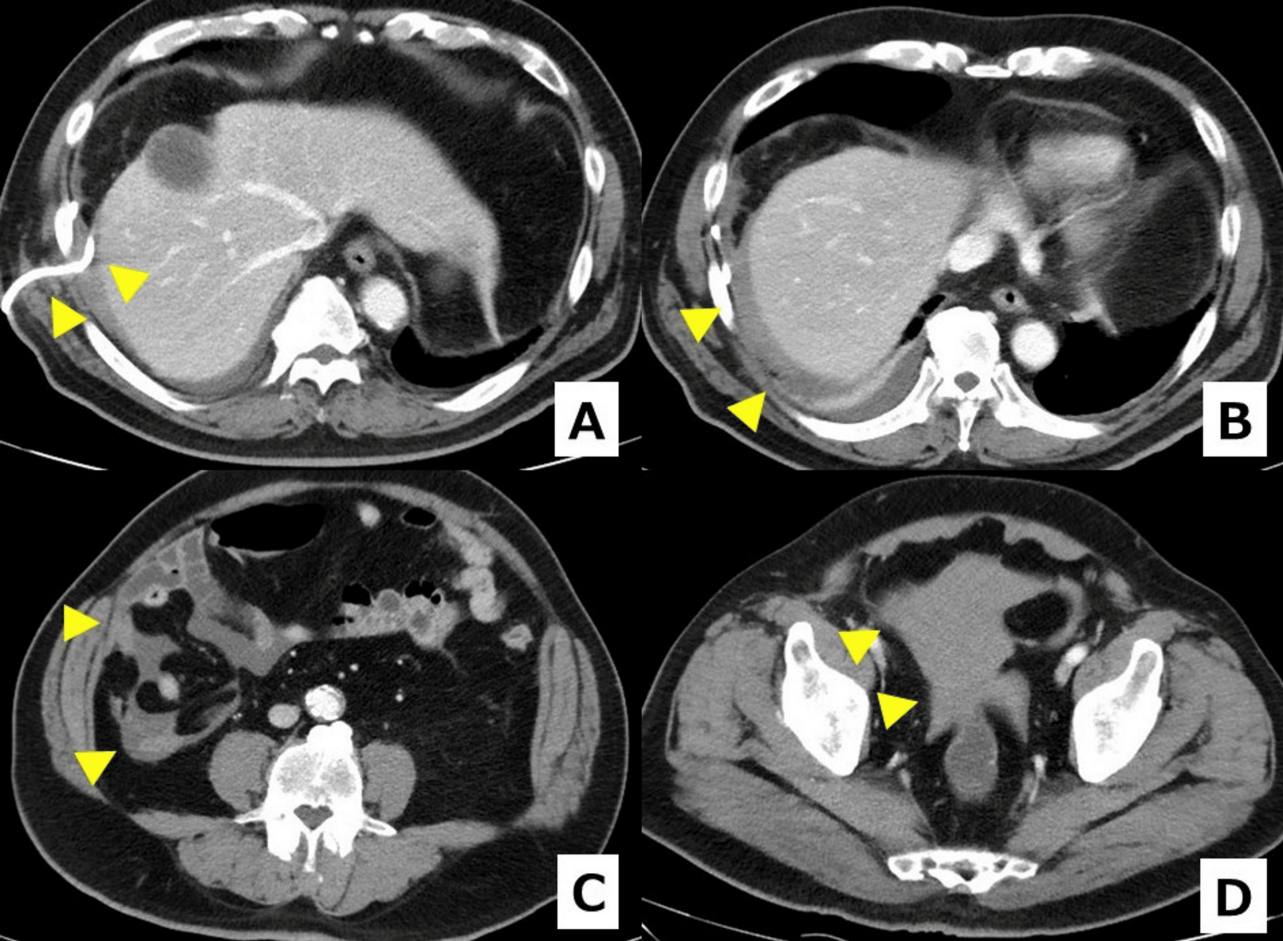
Contrast-enhanced CT after spontaneous PTGBD tube dislodgement (A) Arrowhead: PTGBD tube slipped out of the gallbladder. (B-D) Arrowheads: hemoperitoneum as liquid attenuation bloody ascites layering in the subhepatic space, right paracolic gutter, and pelvis. Attenuation is compatible with acute blood (~30-45 HU), supporting bloody ascites, with no organized hematoma. CT: computed tomography, PTGBD: percutaneous transhepatic gallbladder drainage, HU: Hounsfield units
